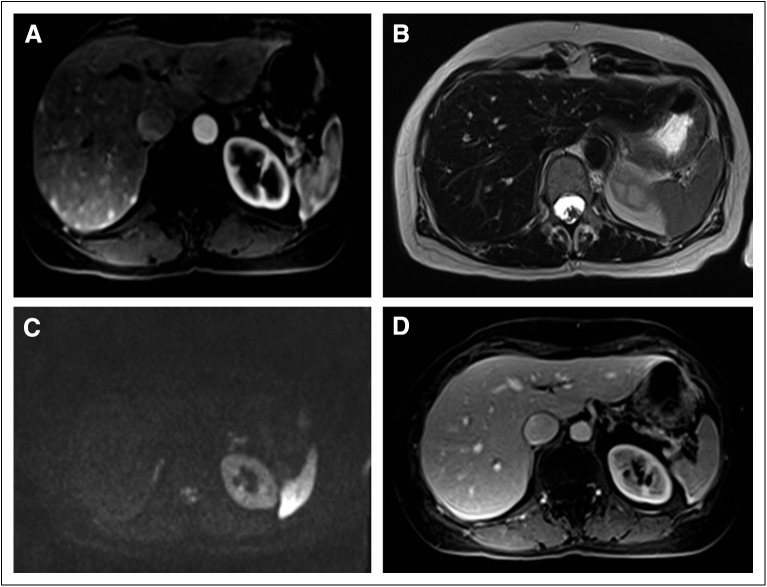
Abdominal magnetic resonance imaging. (A) Three-dimensional gradient echo
